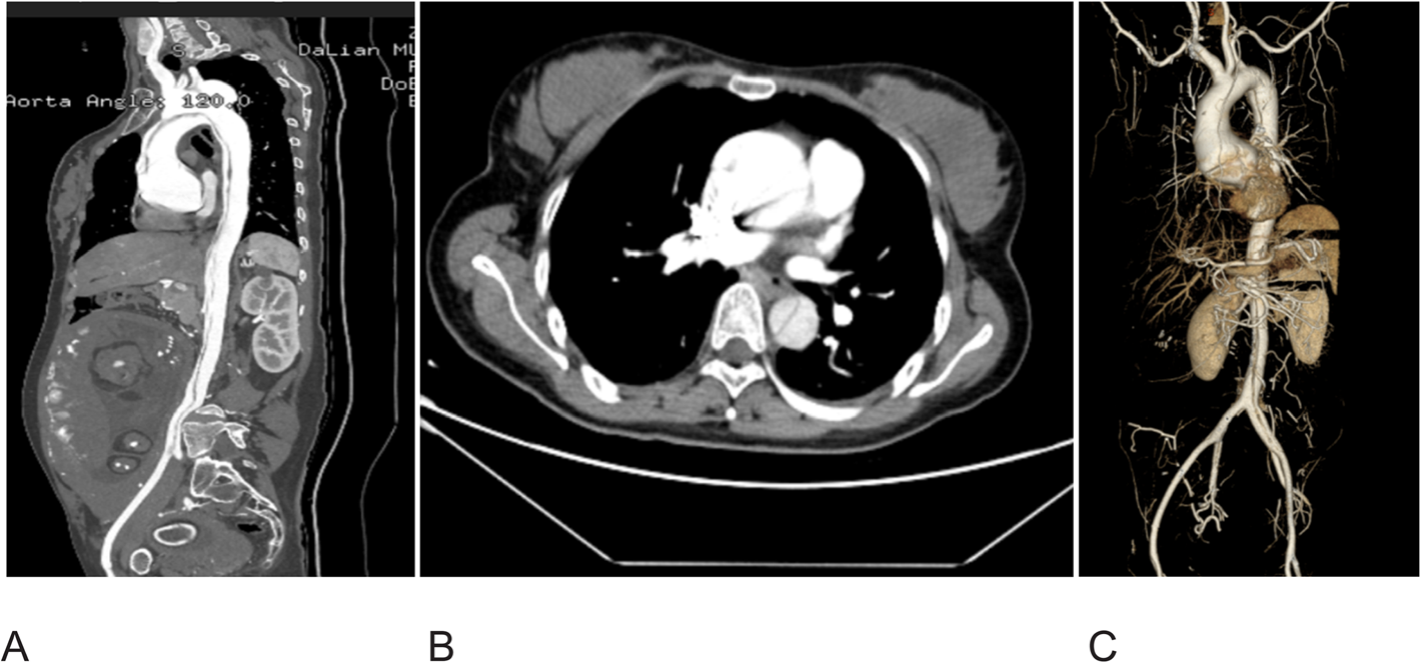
Aortic computed tomography angiograms. a Aortic dissection from ascending aorta to abdominal aorta and the fetus in the uterine cavity. b Acute aortic dissection with a false lumen. c 3-D reconstruction of the aorta, showing dilated aortic sinuses of Valsalva
